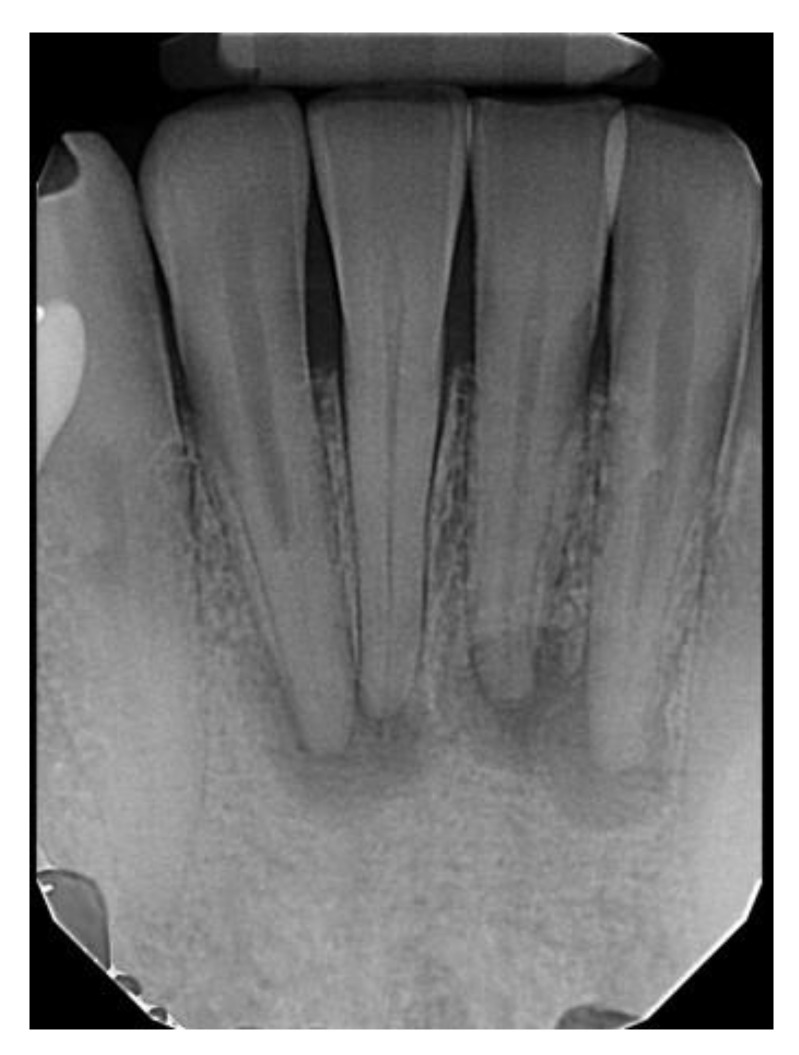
Periapical cemento-osseous dysplasia involving the incisors of the mandible in an asymptomatic 52-year-old Caucasian male.
